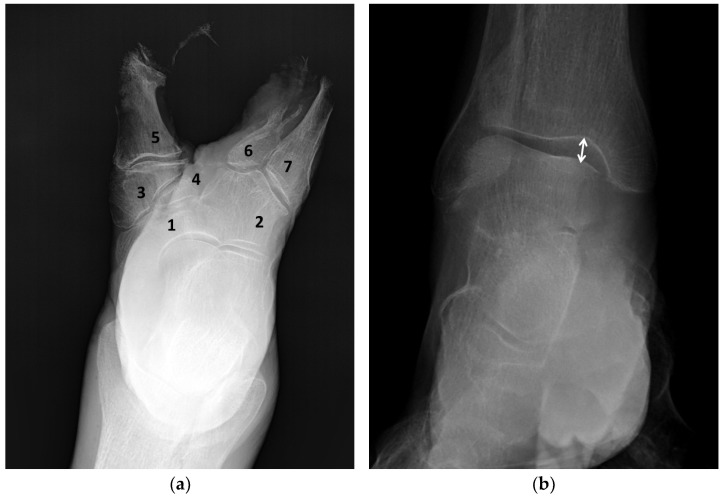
Radiographs of the right foot and ankle: (a)—plantar projection; (b)—anteroposterior projection: 1—navicular; 2—cuboid; 3—medial cuneiform; 4—intermediate cuneiform; 5—first metatarsal; bases and proximal portions of the diaphyses of the fourth (6) and fifth (7) metatarsals; widening of the joint space in the lateral ankle compartment (white arrow).
